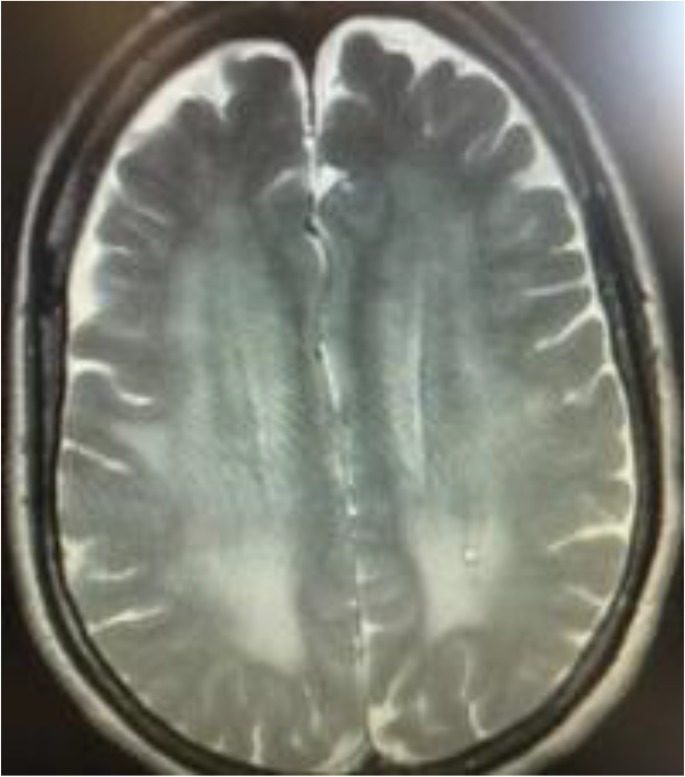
Diffuse hemispheric signal hyperintensity in white matter along the corticospinal tract on axial T2-weighted brain MRI sequence
